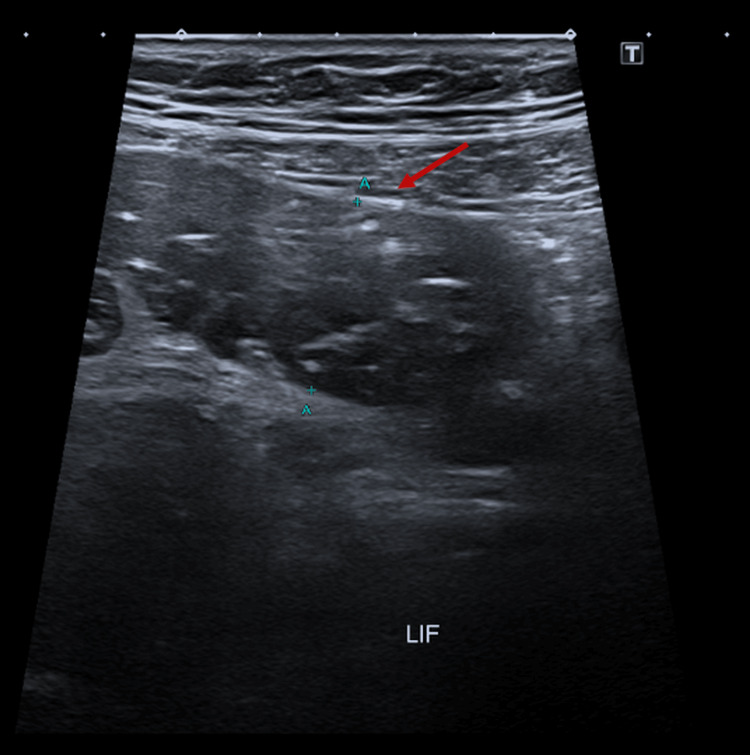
Ultrasound scan of abdomen and pelvis revealing mildly dilated intestinal loops (arrow) measuring around 2.5 cm.
